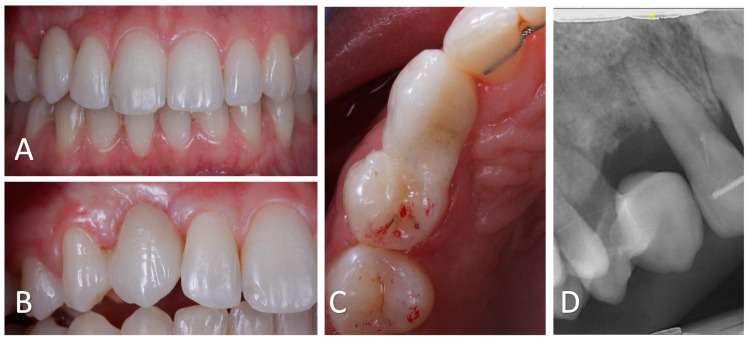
Follow-up at 19 months after surgery. (A): Intra-oral frontal view showing satisfactory aesthetics of the bonded bridge. The black triangle between # 13 and # 12 appears to be smaller. (B). Intra-oral lateral view displaying the healed gingiva in the cervical embrasure on both to the cantilever bridge. (C): Occlusal view of # 13 showing the absence of static or dynamic occlusal charge. (D): Sagittal CBCT radiograph showing bone preservation and partial replacement of the residual root by bone.
